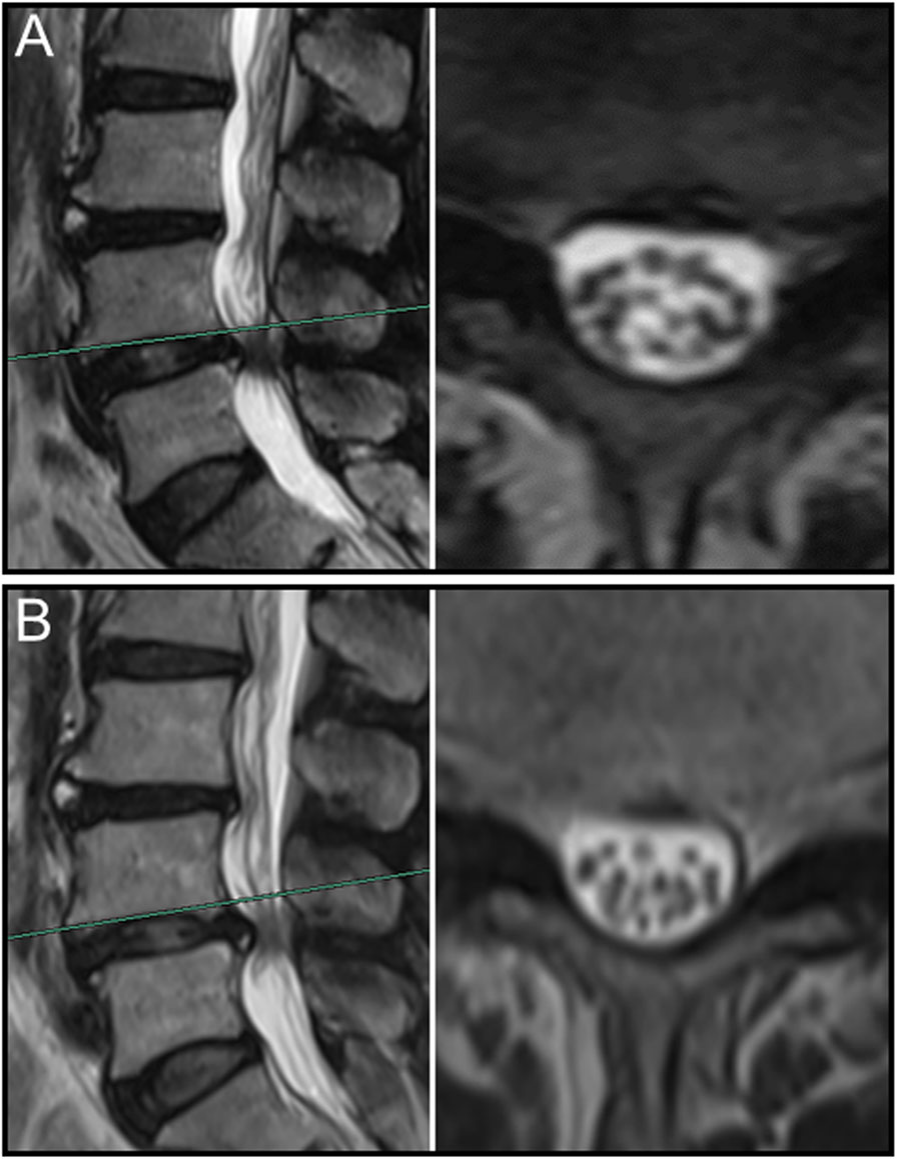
In a woman with positive SedSign and single level spinal stenosis (a), the nerve roots remained stay in the dorsal side of dural sac on prone MR images (b)
